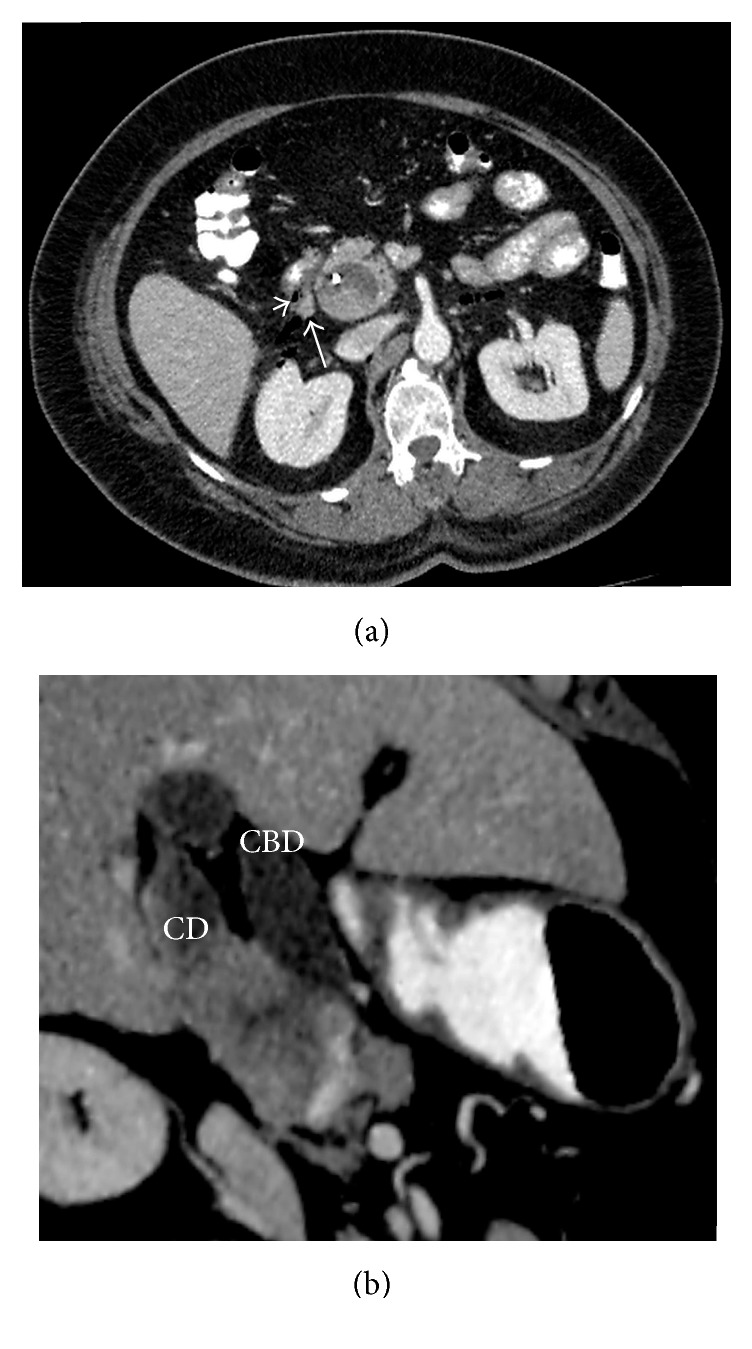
CT examination performed with the suspicion of perforation following ERCP. (a) Axial contrast enhanced CT image shows intraperitoneal free air (short arrow) and periduodenal contrast extravasation (long arrow) suggesting perforation. (b) Multiplanar reconstructed CT image shows fusiform cystic dilatation of the cystic duct having a wide opening to the extrahepatic bile duct which is also fusiformly dilated and the enhancing mass.
